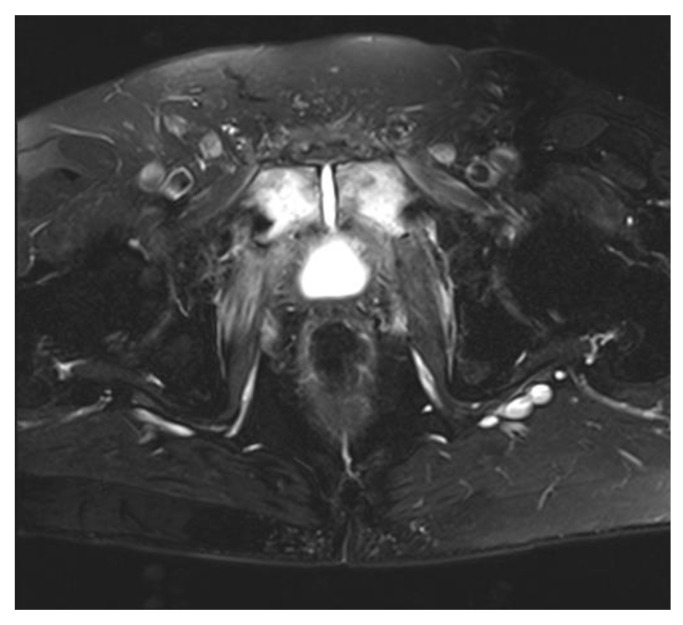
A pelvic MRI of a patient with pubic osteomyelitis. This T2-weighted image is an axial view of the pelvis, which shows an increased signal (bright white) in the bladder as well as the pubic symphysis and surrounding bone consistent with osteomyelitis from a urosymphyseal fistula. Courtesy of Brian Inouye.
